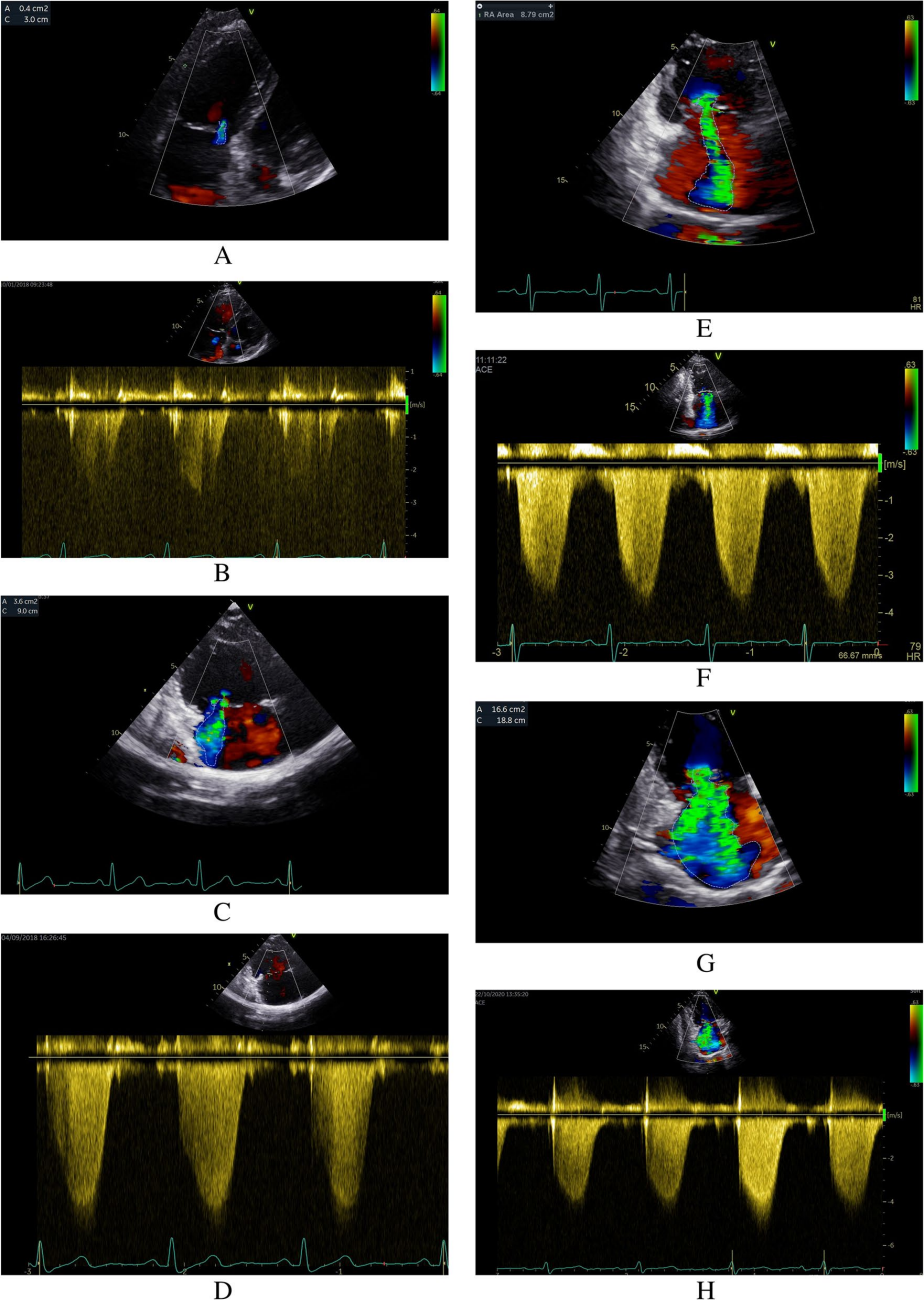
Typical Echocardiography of Color and continuous-wave Doppler in different TR group. A, B: Trace TR group; C, D: Mild TR group; E, F: Moderate TR group; G, H: Severe TR group. TR: tricuspid regurgitation
